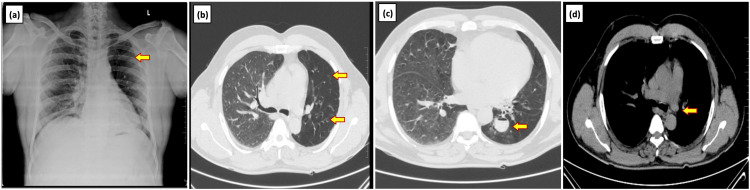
Swyer-James syndrome diagnosed in a 53-year-old male presenting with fever and productive cough (a) Chest radiograph PA view shows unilateral hyperlucency, decreased bronchovascular markings and volume loss of the left lung (yellow arrow). Axial section CT chest (lung window) (b and c) shows diffuse hypoattenuation (arrow) of the left lung and dilated bronchi with the fluid level in the superior segment of the left lower lobe [arrow]. (d) Axial CT chest (mediastinal window) shows reduced caliber of left main pulmonary artery (yellow arrow) PA: Posteroanterior
